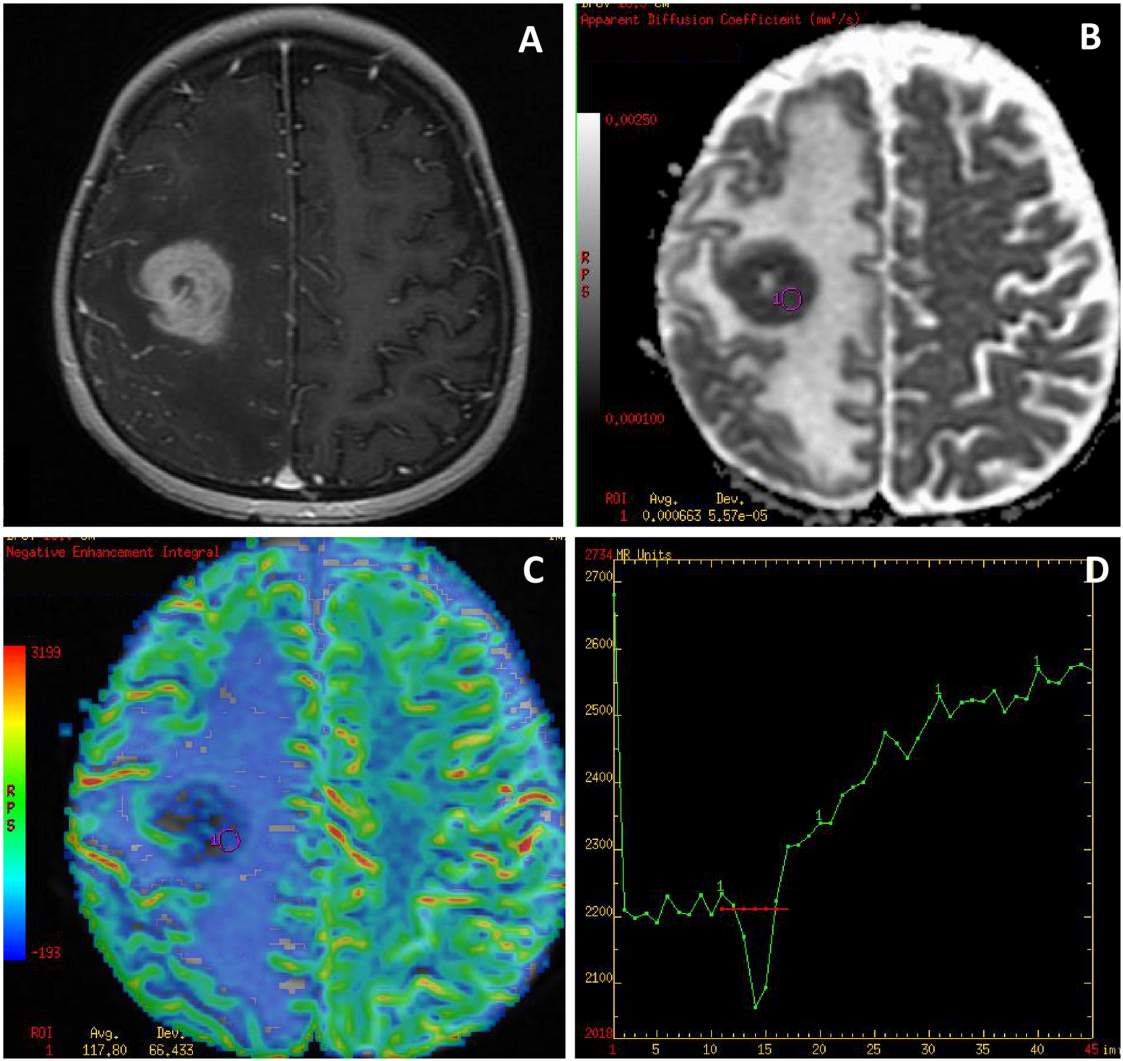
Primary central nervous system lymphoma (PCNSL). (A) a post-contrast T1-weighted image, (B) an ADC map, (C) a CBV map, (D) a perfusion signal intensity time curve. The tumor appears as an enhancing solitary lesion with a large peritumoral edema mimicking a metastasis. The ADC map (B) shows restricted diffusion within the tumor core (min ADC = 0.51 x 10^−3^ mm^2^/s). The CBV map (C) shows the hypoperfused tumor core (max rCBV = 0.79) with the perfusion curve (D) returning above the baseline (red transverse line) which are typical perfusion characteristics of PCNSL.
